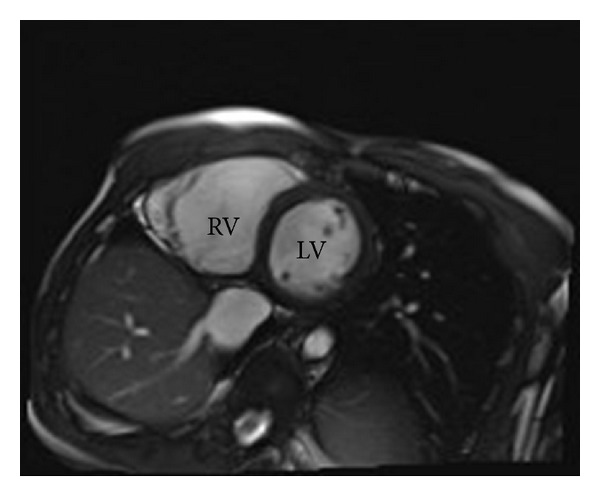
Cardiac magnetic resonance imaging showing right ventricular (RV) enlargement as compared to the left ventricular (LV) size with calculated right ventricular ejection fraction of 35%.
